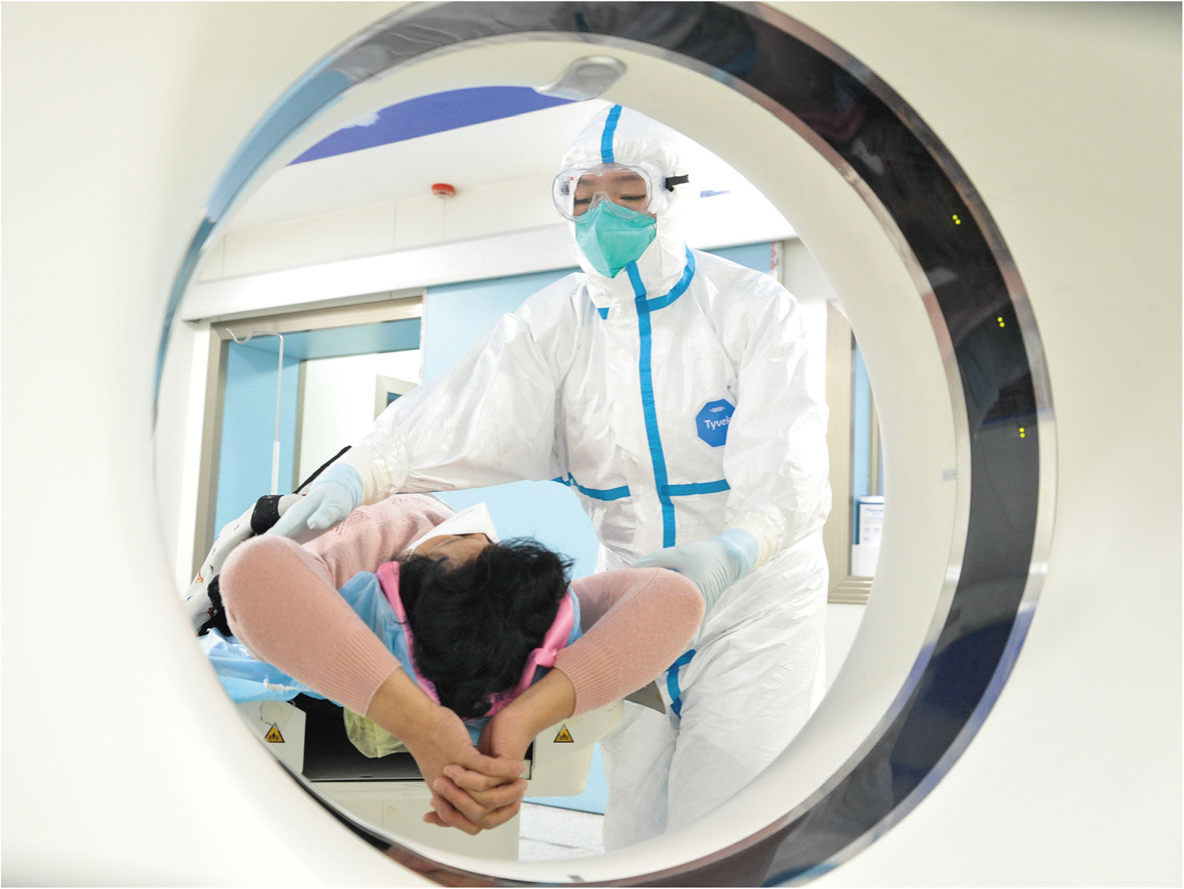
Level 3 PPE during CT examination
